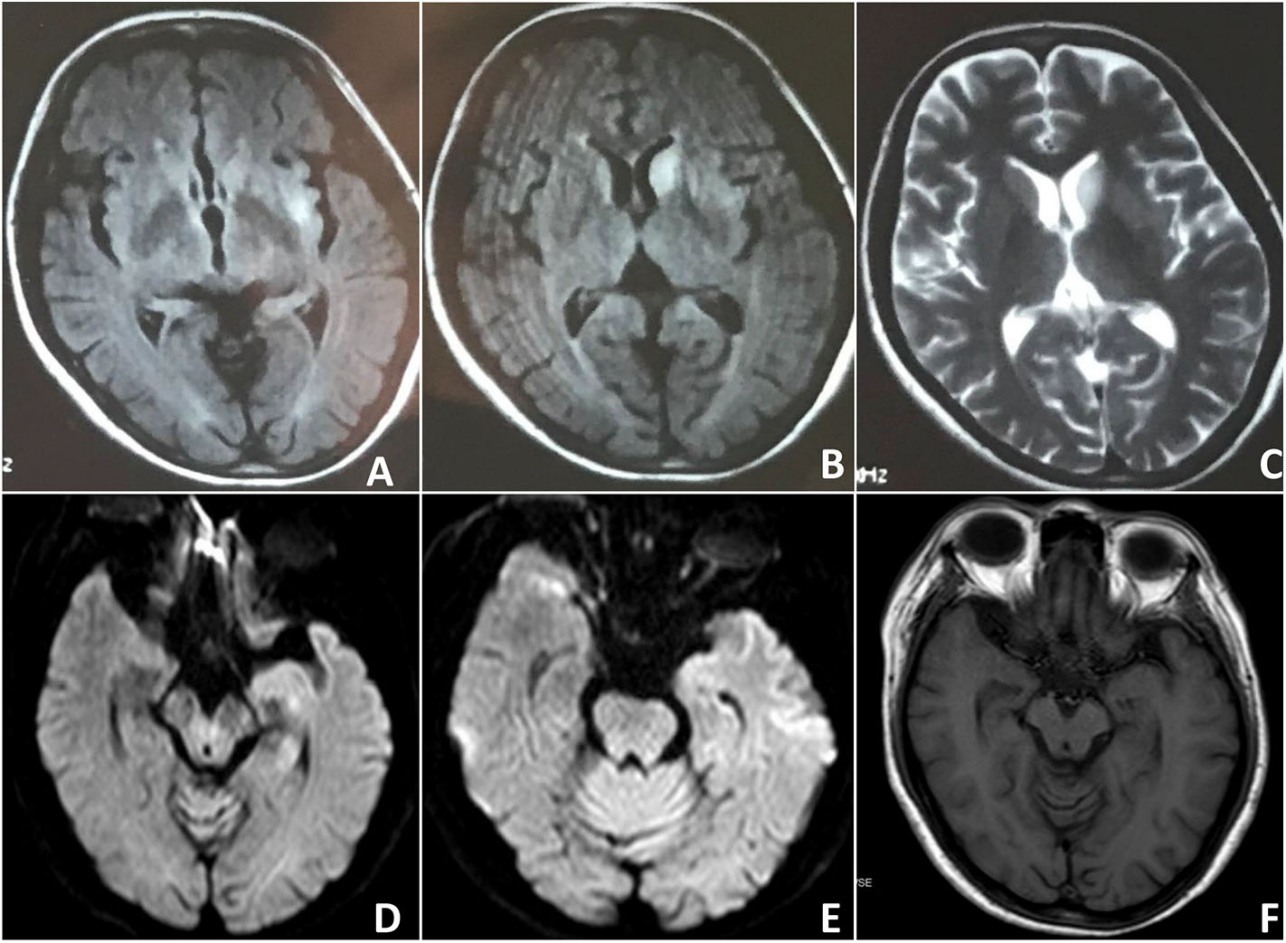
Case 2: Findings on the brain MRI. Initial brain MRI revealed asymmetric FLAIR and T2 hyperintensities in the putamen and caudate. (A–C) Subsequent brain MRI showed bilateral hippocampal volume reduction (D–F).
